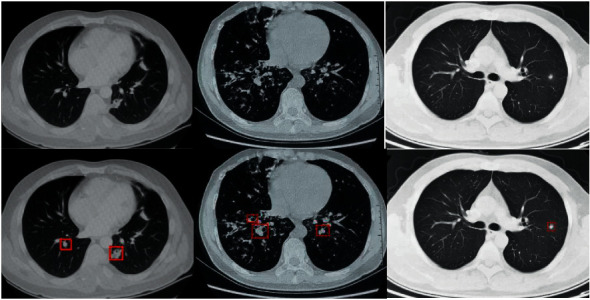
Comparison of the detection effect of R-FCN algorithm on CT images of pulmonary nodules (the box represents the automatic segmentation labeling of pulmonary nodules by R-FCN algorithm).
